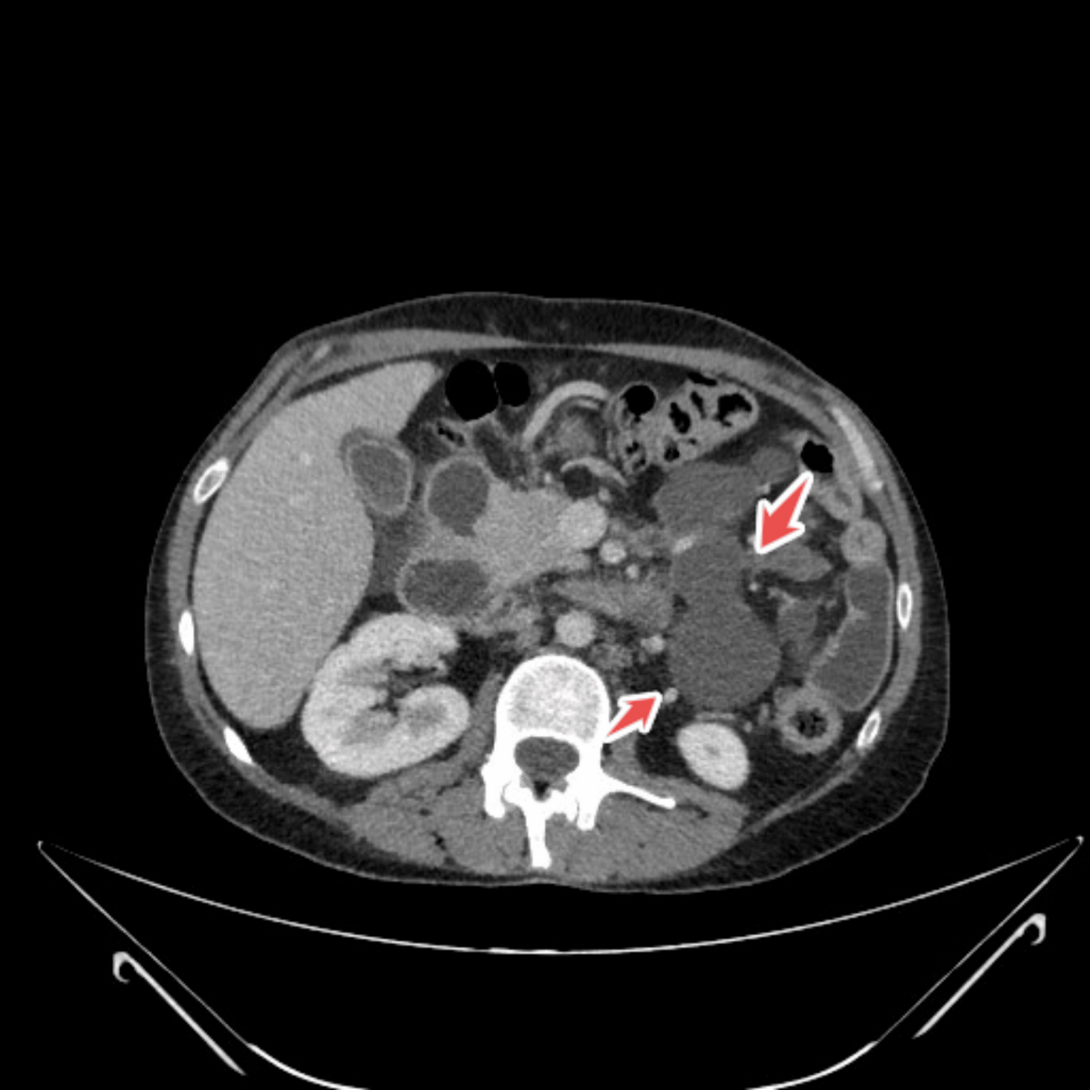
CT abdomino-pelvic showing loculated cystic structures in the left upper quadrant highlighted by arrows
